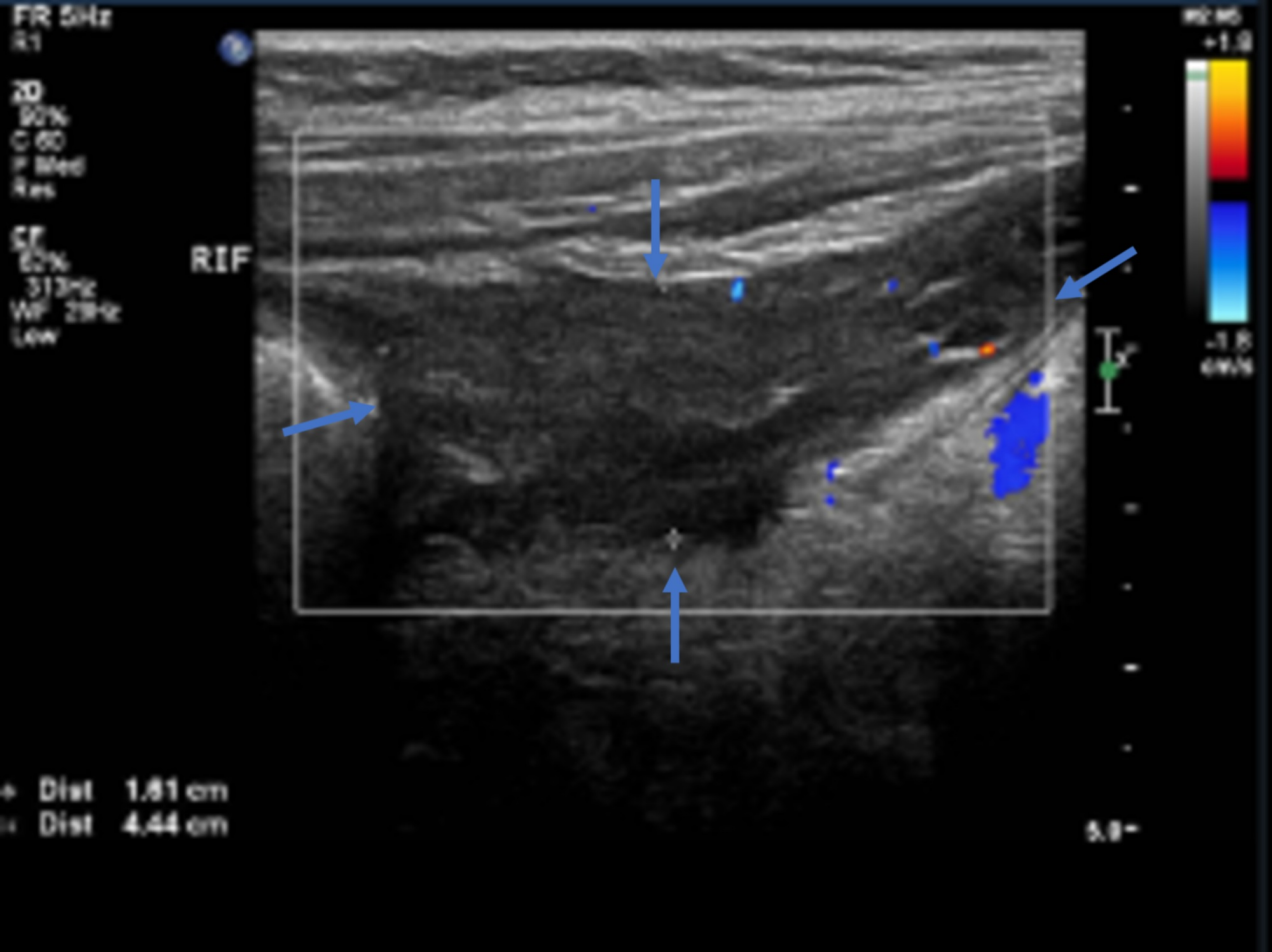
Ultrasonography of the abdomen - view 2 The image shows adjacent turbid collection (arrows) measuring 1.6 × 4.4 cm, indicating perforated appendicitis
